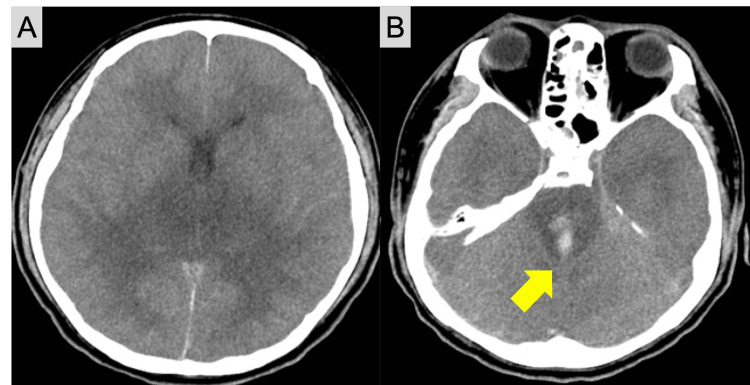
Head computed tomography (CT) scan after the resuscitation The findings of the head CT scan after the resuscitation demonstrated diffuse cerebral edema (A) and intracranial hemorrhage (arrow) in the brain stem, which expanded to the fourth ventricle (B).
